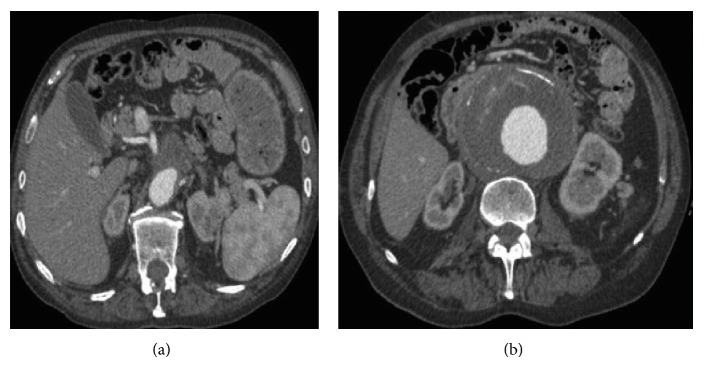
Preoperative CTA showing the extension of the AAA to the juxtarenal tract of the abdominal aorta (a) and the maximum diameter of the aneurysm (b). No signs of hydronephrosis were noted preoperatively.
